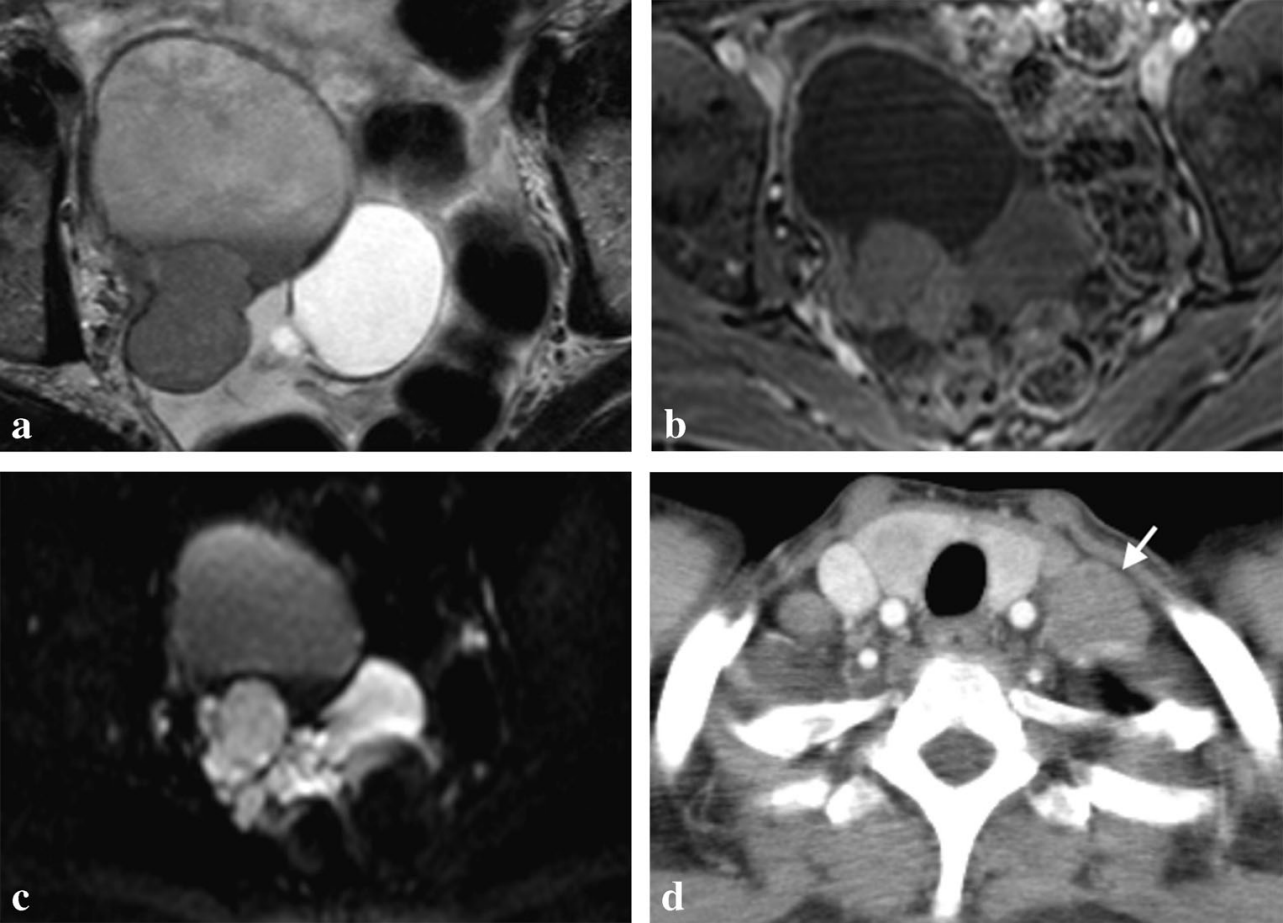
a-d. High-grade serous carcinoma of the right ovary in a 56-year-old BRCA1-mutation carrier woman. Magnetic resonance imaging (MRI) scan with T2-weighted (a), post-gadolinium fat-suppressed (FS) T1-weighted (b) and diffusion-weighted (c) images, showing a complex cystic-solid multilobulated right adnexal mass with restricted diffusion on b600 image (c), with pathologically proved capsule rupture, but without metastases. Contrast-enhanced computed tomography (CECT) obtained 1 year later due to a left supraclavicular palpable painful mass confirms the presence of a lymph node metastasis (*arrow* in d)
